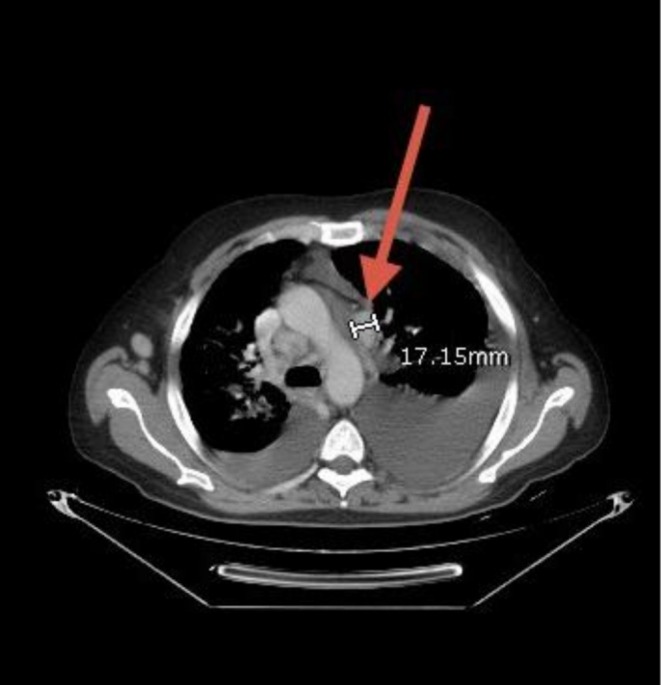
Mediastinal lymphadenopathy. Contrast‐enhanced CT showing multiple enlarged mediastinal lymph nodes, consistent with thoracic involvement in systemic disease.
